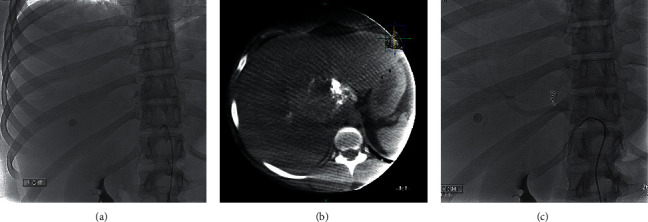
(a) Subselective catheterization of the hepatic artery, segment 8 branch with arteriography. (b) Cone beam CT demonstrating patency and perfusion of the hepatic adenoma. (c) Coil embolization of segment 8 branch of the hepatic artery.
